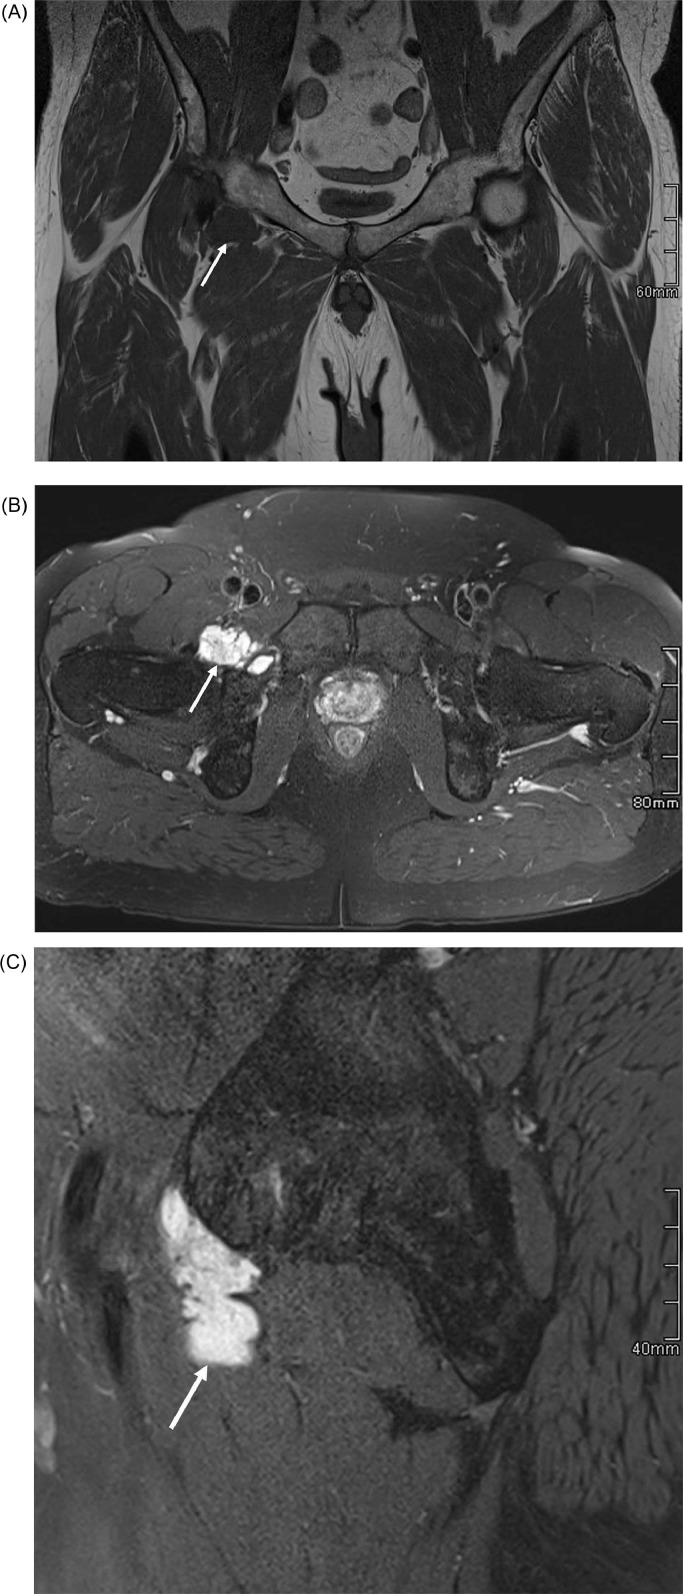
Selected pelvic MRI slices of the same patient. (A) Coronal T1 imaging demonstrates a homogenous low T1 signal lesion (white arrow) anterior to the right hip joint. (B) Axial proton density fat-saturated and (c) sagittal proton density fat-saturated slices demonstrate a lobulated high signal lesion (arrows) close to the hip joint extending into the area of the obturator externus bursa.
